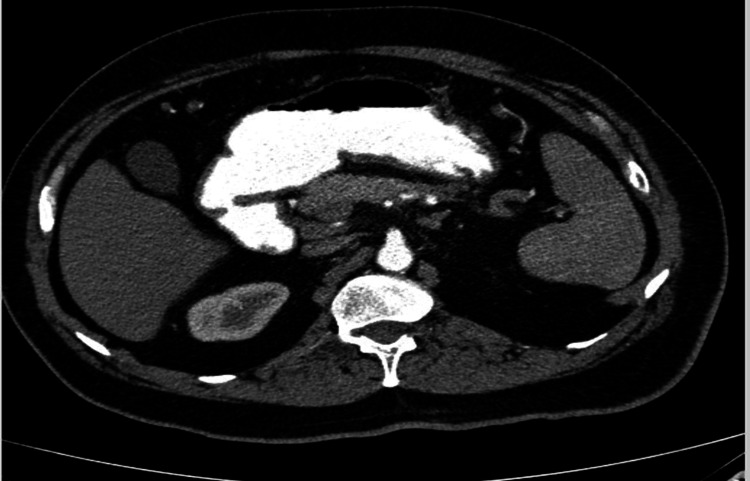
CT abdomen after treatment (axial view) The CT showed complete resolution of pancreatic lesions.
